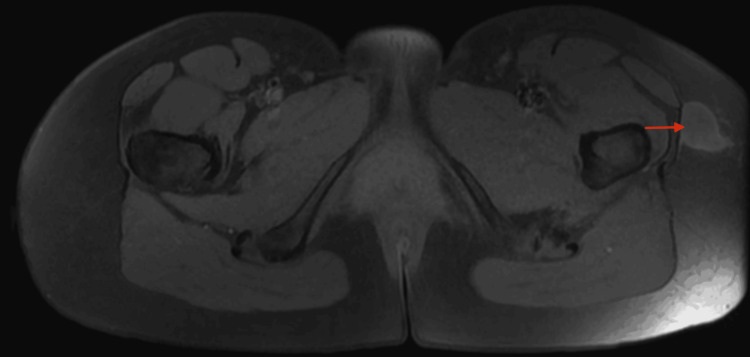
Axial non–contrast-enhanced T1-weighted fat-suppressed pelvic MRI shows a well-circumscribed superficial soft-tissue nodule along the lateral aspect of the left hip, adjacent to the tensor fasciae latae (red arrow). The lesion remains isointense to skeletal muscle and shows no intralesional fat, with preserved surrounding planes and no adjacent bone involvement.
